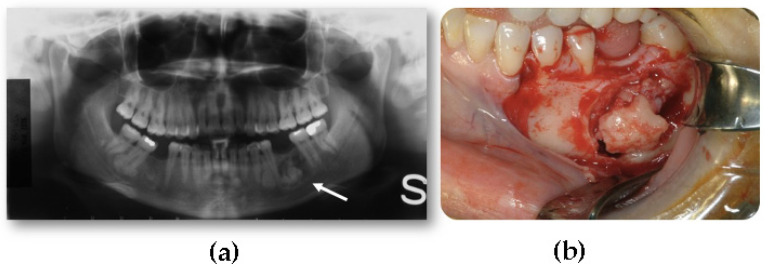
A case of a mandibular osteoma. (a) The panoramic radiograph demonstrates a focal radiopaque lesion (white arrow); (b) Osteoma’s surgical removal via the transoral approach at).
